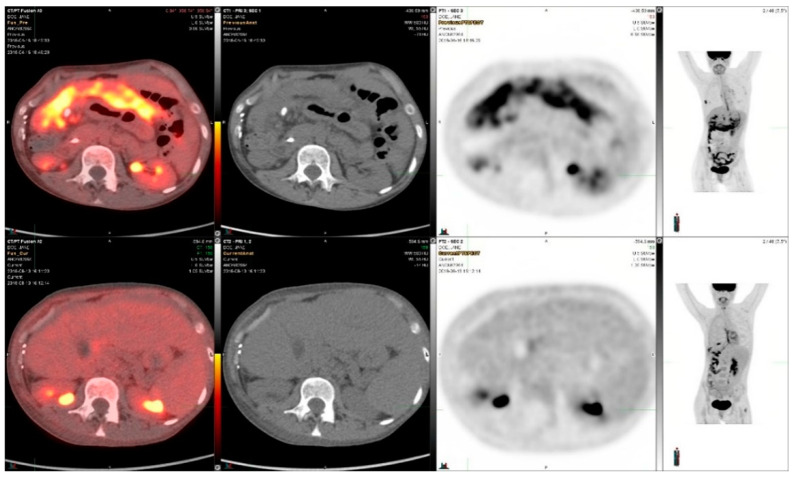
^18^F-FDG-PET/CT images in a patient with metastatic adenocarcinoma of the pleura which had an RET-mutation and was treated with selpercanitib for 6 cycles. The upper panel demonstrates the situation before selpercanitib treatment; from the left, transaxial PET/CT fusion images, CT images, and PET images in the upper abdominal region and the furthest right image shows an MIP image from the whole-body PET study. The lower panel shows the situation after two cycles of selpercanitib two months later in images at the same level in the transaxial images and in the MIP image furthest to the right. In this metastatic area the SUVmax decreased from 8.2 to 2.1, corresponding to a 74% decrease.
